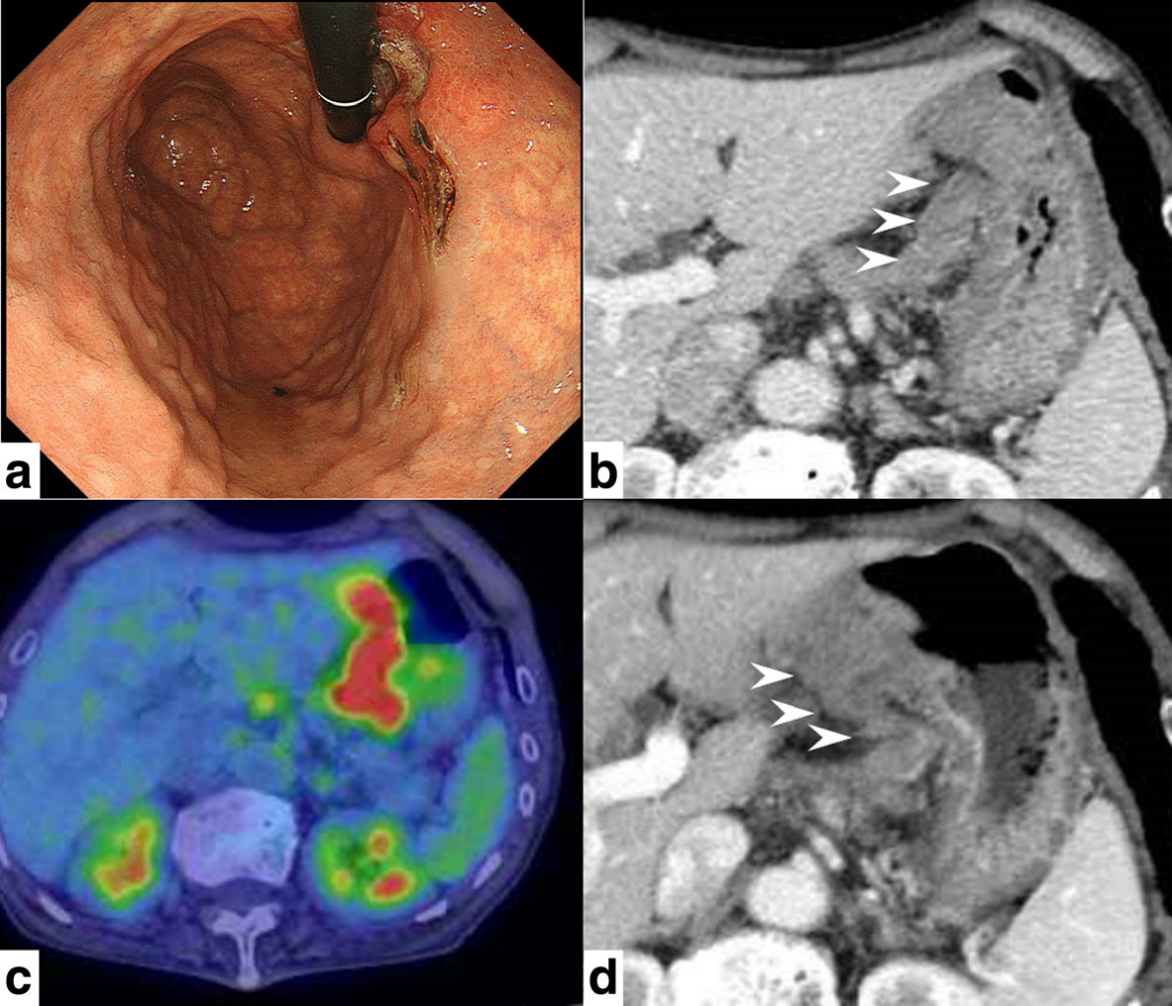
a Endoscopic findings. A Borrmann type III tumor was found in the cardiac end of the stomach and irregular wall thickening was identified in the lesser curvature. b Abdominal contrast-enhanced computed tomography (CT) findings. Abdominal contrast-enhanced CT indicated that the lesser curvature stomach wall was modestly enhanced with suspicious bulky lymph nodes in the lesser curvature (white arrowhead). c Positron emission tomography (PET) findings. PET revealed an accumulation of fludeoxyglucose (18F) in the stomach wall and bulky lymph nodes were detected by computed tomography (CT). d Abdominal contrast-enhanced computed tomography (CT) findings after preoperative chemotherapy. Abdominal contrast-enhanced CT demonstrated that the size of tumor and bulky lymph nodes had not changed (white arrowhead)
